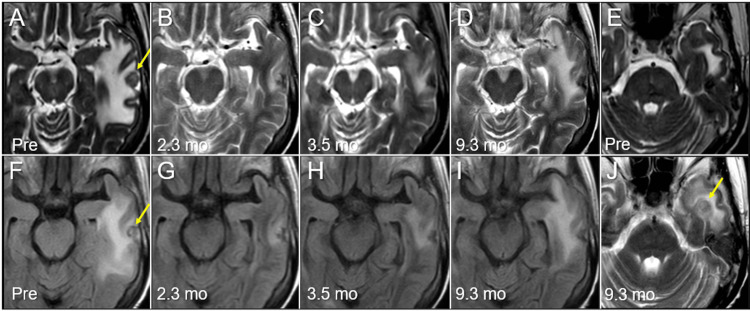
Case 2: Magnetic resonance images before and after five-fraction stereotactic radiosurgery The images show axial T2-WI (A-E, J); axial FLAIR images (F-I); before SRS (Pre) (A, E, F); at 2.3 months (mo) after SRS (B, G); at 3.5 months (C, H); and at 9.3 months (D, I, J). (A) A mass lesion (arrows in A, F) in the left temporal lobe is associated with perilesional edema. (B-D, G-I) The tumor shrinkage is observed at 2.3 months, and the low-intensity tumor remnant remained stable at 9.3 months. The perilesional edema significantly decreased at 2.3 months with a subsequent gradual increase. (J) A new lesion is observed in the left temporal base (arrow in J), which contributes partly to the aggravation of the perilesional edema in the left temporal lobe. WI: weighted image; FLAIR: fluid-attenuated inversion recovery; SRS: stereotactic radiosurgery
